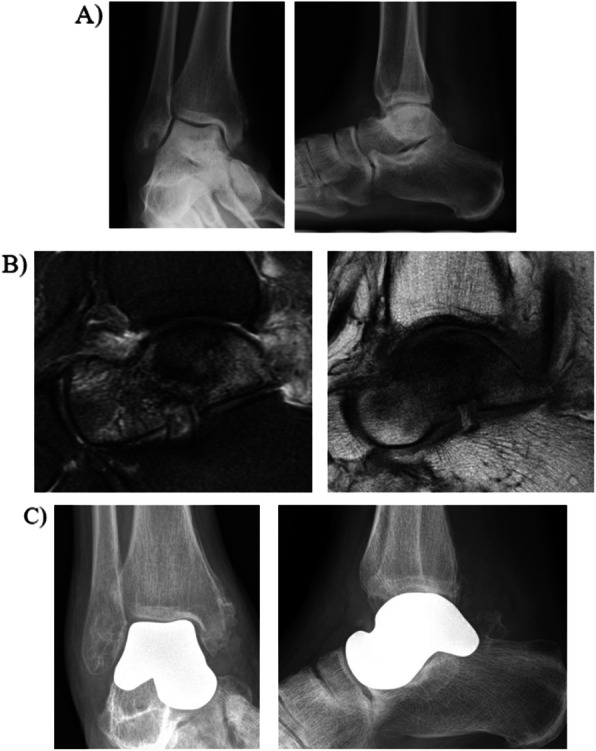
Total talus arthroplasty for talar avascular necrosis. a AP and lateral radiographs demonstrate significant sclerosis of the talar body with some central collapse b Sagittal T2 and T1 cuts demonstrating diffuse talar avascular necrosis. c Total talus arthroplasty with custom 3D printed cobalt chrome prosthesis. Implant is designed based on imaging from the contralateral normal talus
